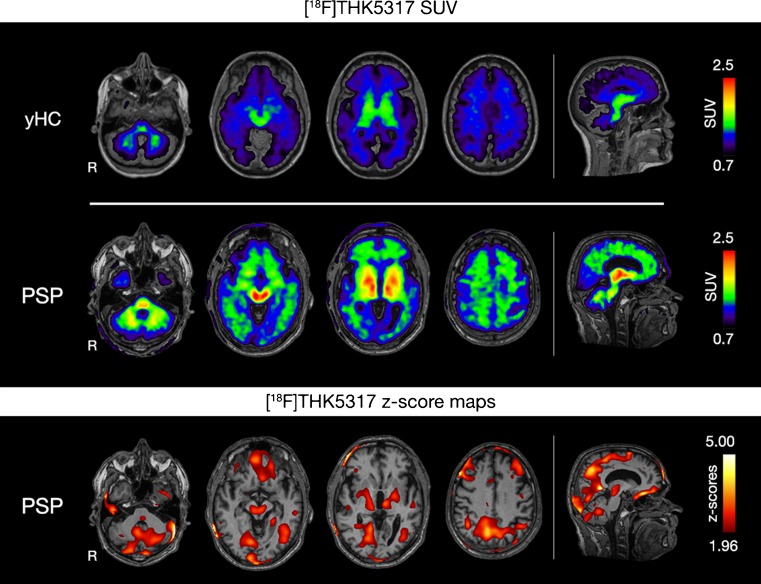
[^18^F]THK5317 SUV retention in a representative young healthy control (yHC) and a patient with progressive supranuclear palsy (PSP) (upper two rows), [^18^F]THK5317 SUV z-score map in comparison to yHCs for the PSP patient (last row). A z-score threshold of 1.96 (95 % confidence interval) was applied. R = Right; SUV = standard uptake value as the radioactivity concentration (MBq/mL) divided by [injected dose (MBq)/ patient’s weight (kg)].
